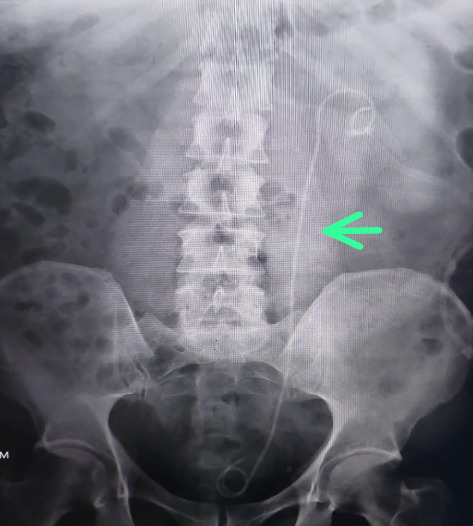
Post-op x-ray showing left stone free with DJ stunt in situ.
